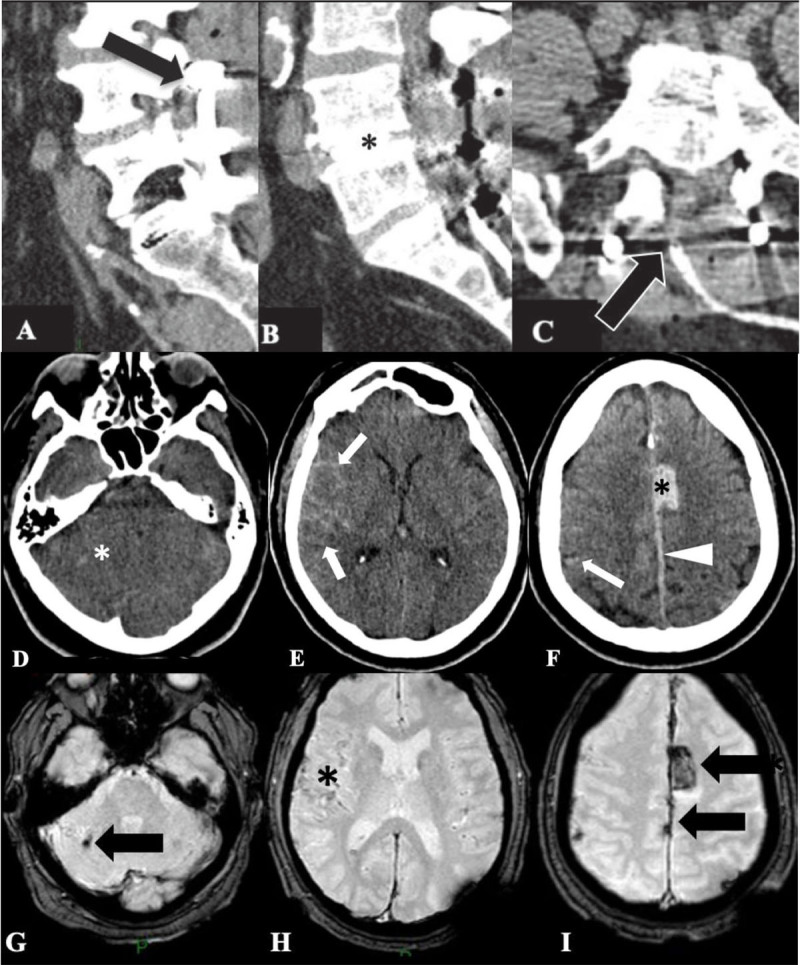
Patient 2. (A, B) Sagittal lumbar CT images show the L4-L5-S1 posterior arthrodesis (*arrow*) and implementation of L4-L5 disc prosthesis (*); (C) Axial CT image at the L5-S1 level shows a catheter at the site of the laminectomy (*arrowhead*). (D–F): Axial brain CT images show a tiny right cerebellar hematoma (D, *), diffuse subarachnoid hemorrhage (E, F, *arrows*), a left interhemispheric subdural hematoma (F, *arrowhead*) and a parasagittal left superior frontal intraparenchymal hematoma (F, *).
